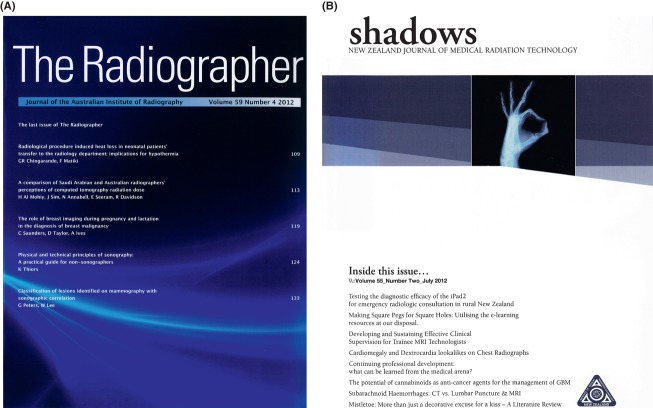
(A) 2012 cover page of *The Radiographer* and (B) 2012 cover page of *Shadows*.
